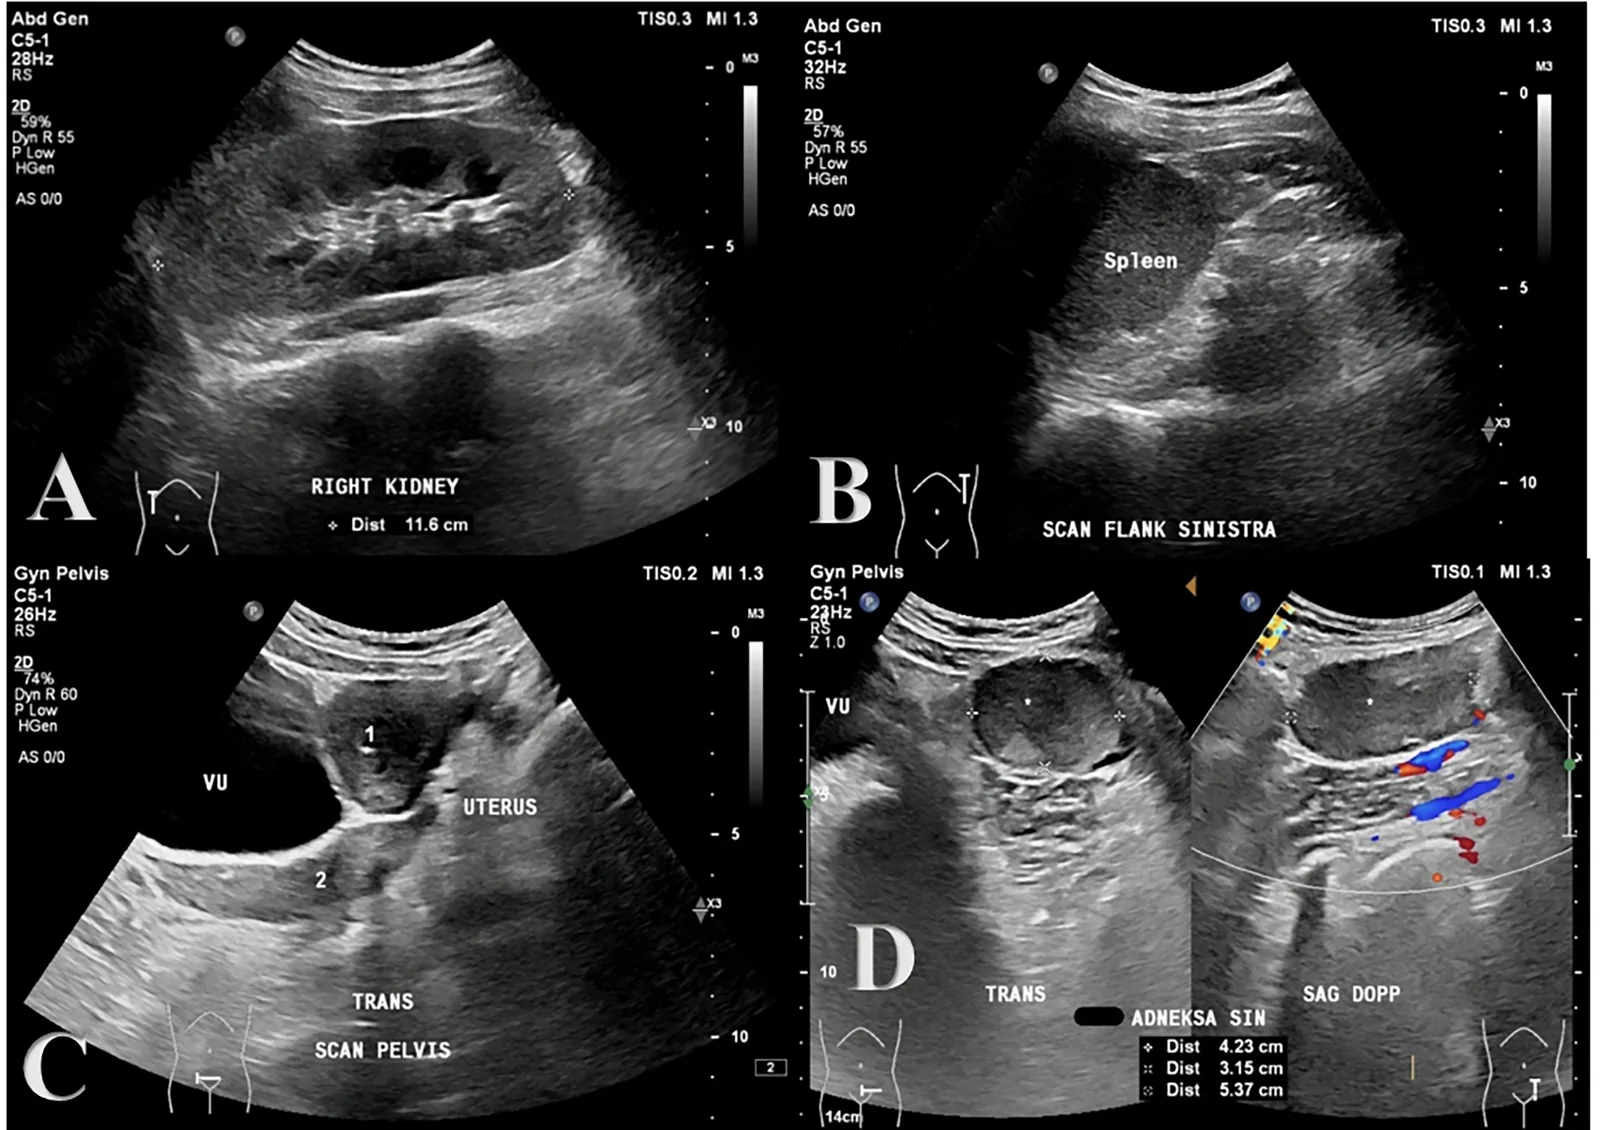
Transabdominal ultrasonography findings. (A) Normal right kidney. (B) Absence of the left kidney, consistent with renal agenesis. (C) Two separate uterine structures, suggestive of uterus didelphys. (D) Left adnexal cystic lesion demonstrating homogeneous low-level internal echoes with a ground glass appearance and peripheral echogenic foci, consistent with an endometrioma.
